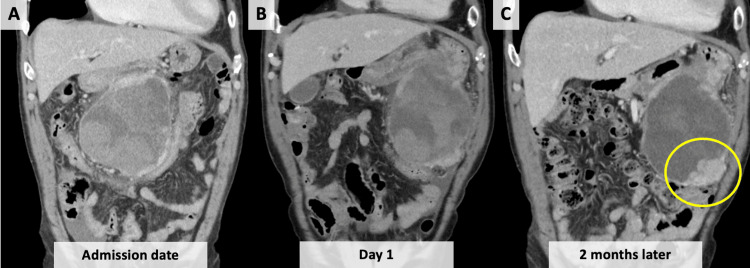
The course of contrast-enhanced abdominal CT scan findings revealing the size of the hematoma did not change. (A) Admission date. (B) Day 1. (C) Two months later. A part of the caudal side of the hematoma had a high-density area that had not been seen before (yellow circle). CT: computed tomography
